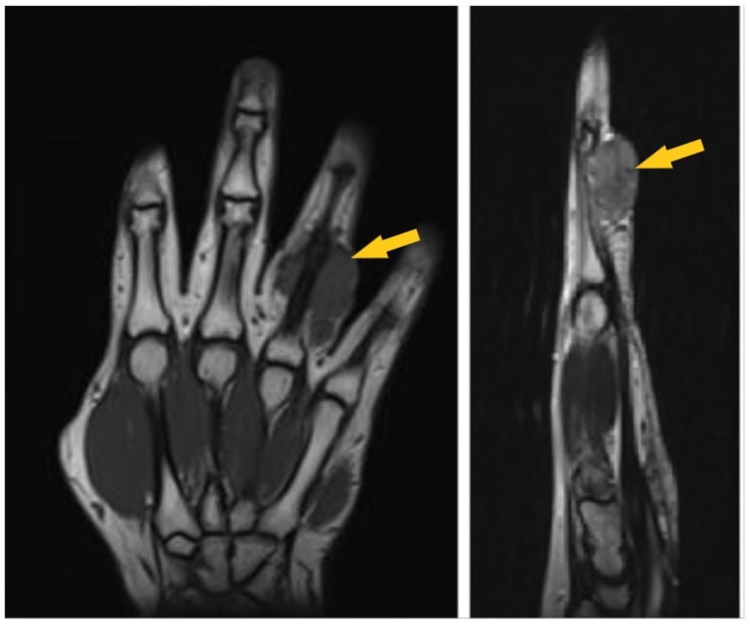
MRI (left: axial cut section; right: sagittal section) showing tumor in the right fourth finger with compression of the ulnar neurovascular bundle (yellow arrow).
